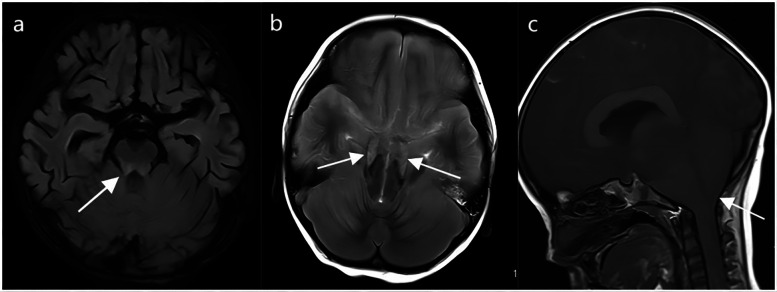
The cranial MRI in fatal cases of HFMD. (a) Abnormal brain stem signals from brain stem encephalitis in HFMD. (b) Tentorial hernia in HFMD. (c) Cerebellotonsillar hernia in HFMD. The arrow points to the lesion.
